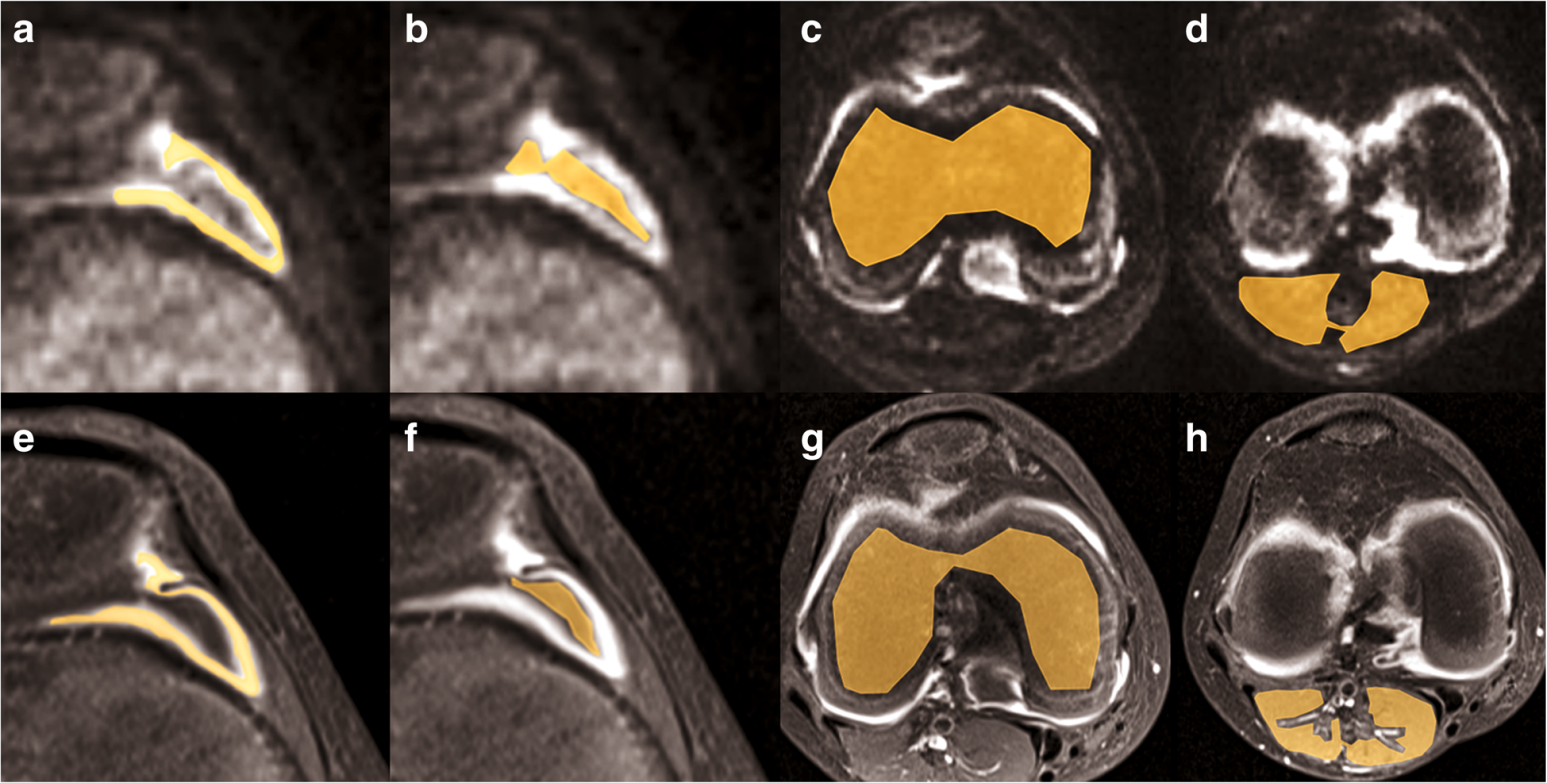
Same patient as in Fig. 1. Region of interest (ROI) placement using half-translucent polygonal ROIs on DWI b = 800 s/mm^2^ (a-d) and contrast-enhanced MRI (e-h) for manual segmentation of synovium (a, e), joint effusion (b, f), femoral epiphyseal bone marrow (c, g) and upper calf muscle (d, h)
